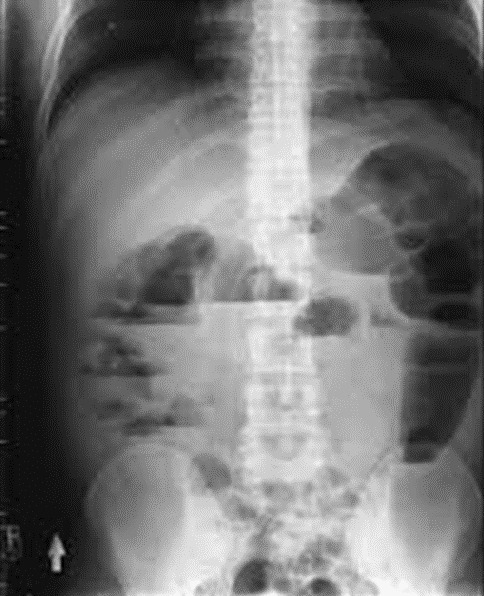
Erect plain abdominal Xray showing multiple air-fluid levels from advanced PCa
